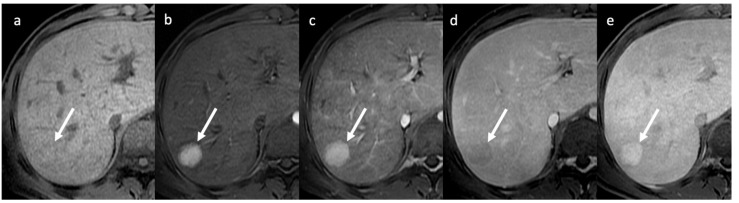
MRI with hepatospecific contrast agent shows a regenerative nodule (arrows) in chronic BCS, isointense on pre-contrast T1 (a), markedly and homogeneously hypervascular on arterial and portal venous phases (b,c), and with wash-out on delayed phase (d) mimicking HCC. On hepatobiliary phase (e) the lesion appears homogeneously hyperintense to normal liver; this helps to differentiate FNH-like regenerative nodule from HCC.
